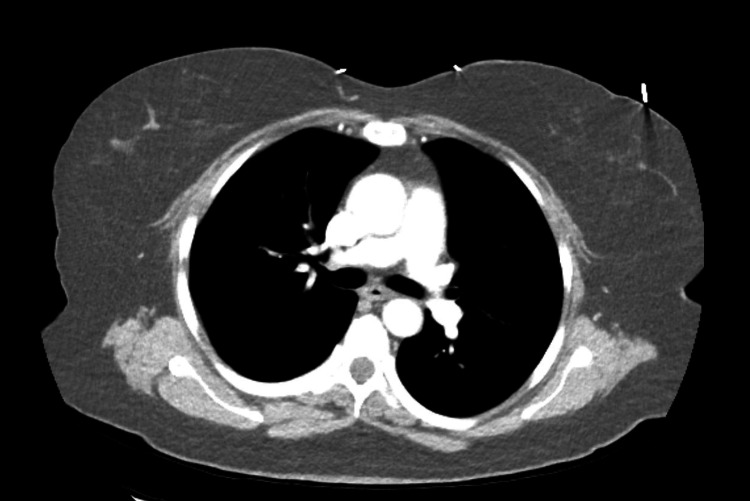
CT angiography of the chest demonstrating no evidence of pulmonary embolism.
